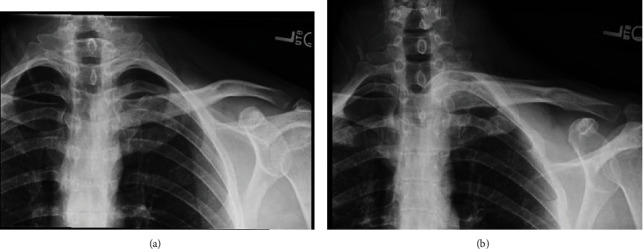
(a, b) Plain radiographs demonstrating anterior dislocation of the sternoclavicular joint as well as a posterior acromioclavicular dislocation.
